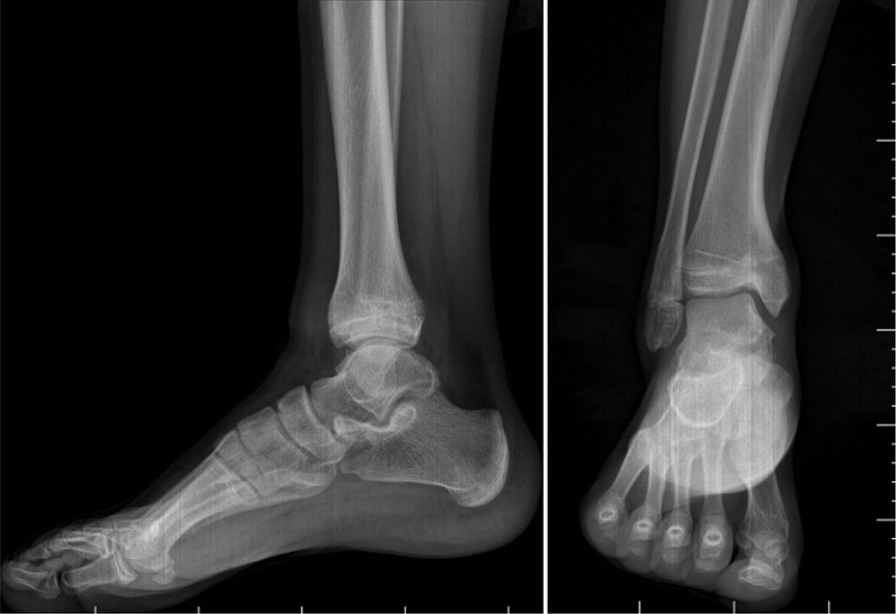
Two-year post-operative X-ray
